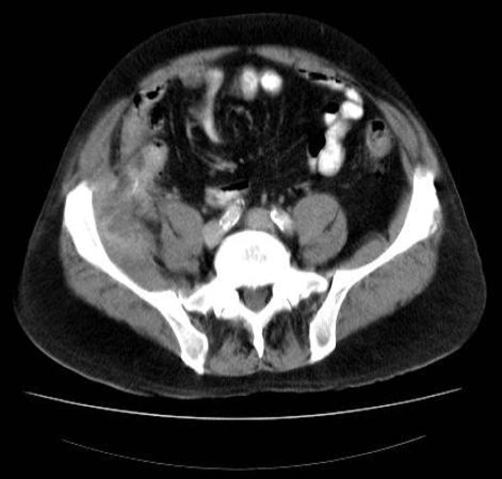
Repeat CT scan of the abdomen a few months later showing the same foreign body embedded in iliopsoas muscle with an abscess in the area.
